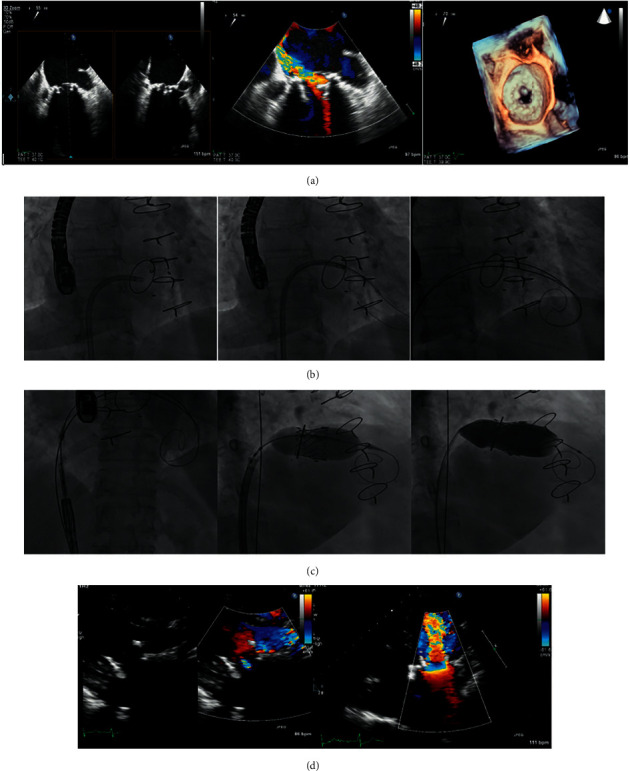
Transcatheter mitral valve in a degenerated Hancock™ II (Medtronic) valve (TMViV). A 66-year-old diabetic male patient, with ulcerative colitis, had a degenerated bioprosthetic 29 mm Hancock™ II MV with an associated AF. TEE and 3D-TEE show a degenerated bioprosthetic 29 mm Hancock™ II MV (severe MS and severe MR). (b) Fluoroscopy shows an 8.5F-agilis™ sheath was flexed and directed towards the degenerated bioprosthetic MV. A 5F-MP catheter over a 0.035-inch curved Terumo guidewire crossed the MV to the LV. The wire was exchanged with a 0.035-inch/260 extra-stiff Confida™ guidewire and a 0.035-inch/260 J-curve super-stiff Amplatz™ guidewire. (c) Fluoroscopy shows TMViV implantation of an Edwards SAPIEN-3 26 mm valve within the degenerated bioprosthetic MV, followed by post-TMViV distal valve flaring. D: TTE: an Edwards SAPIEN 3 26 mm valve in the MV position with normal Pg, and trivial with a valvular leak. AF: atrial fibrillation, 3D: three-dimensional, MR: mitral regurge, MS: mitral stenosis, MV: mitral valve, MVA: mitral valve area, Pg: pressure gradient, TMViV: transcatheter mitral valve-in-valve, TEE: transesophageal echocardiography, TTE: transthoracic echocardiography.
